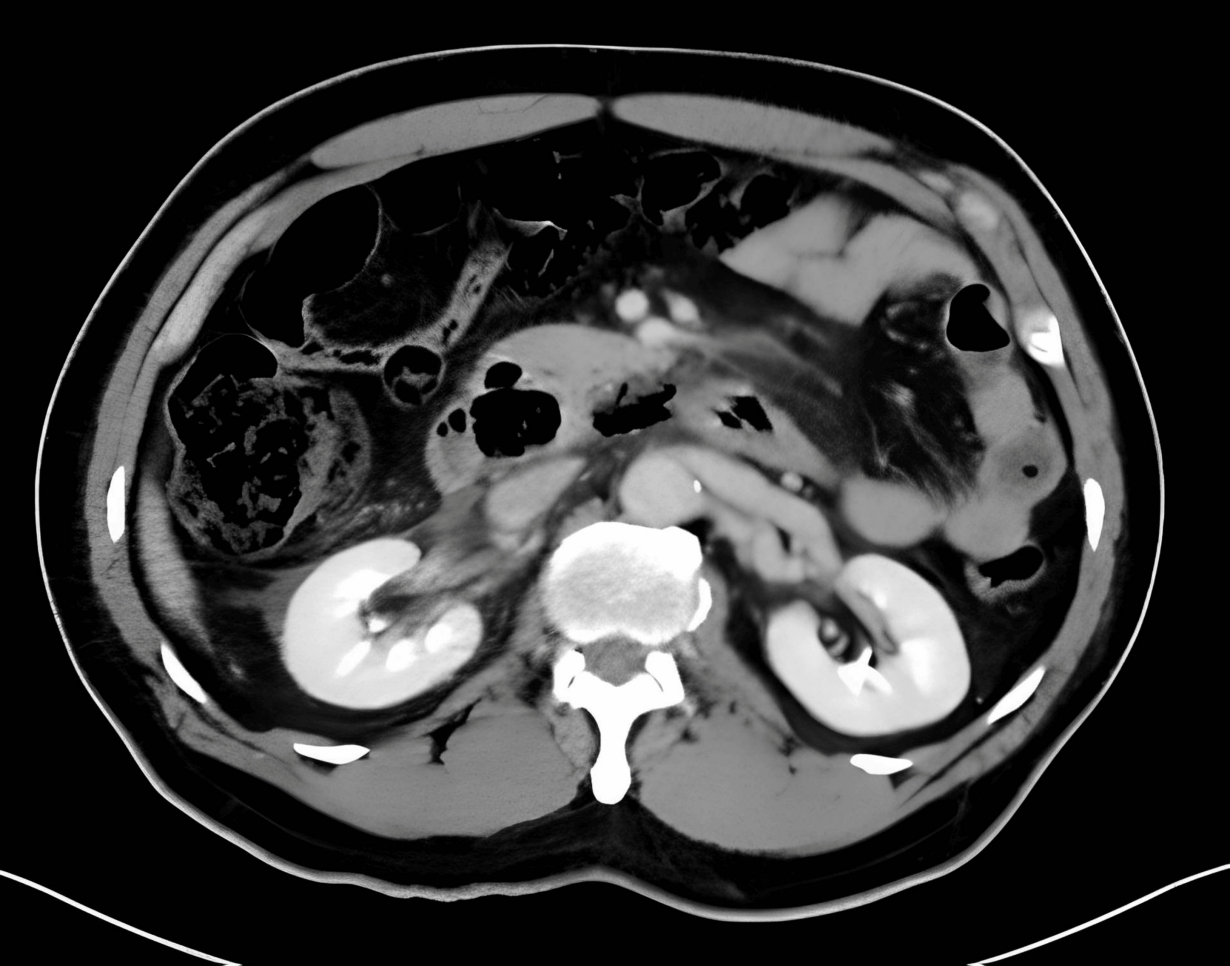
CT film demonstrating perforation of duodenum with retroperitoneal free gas
